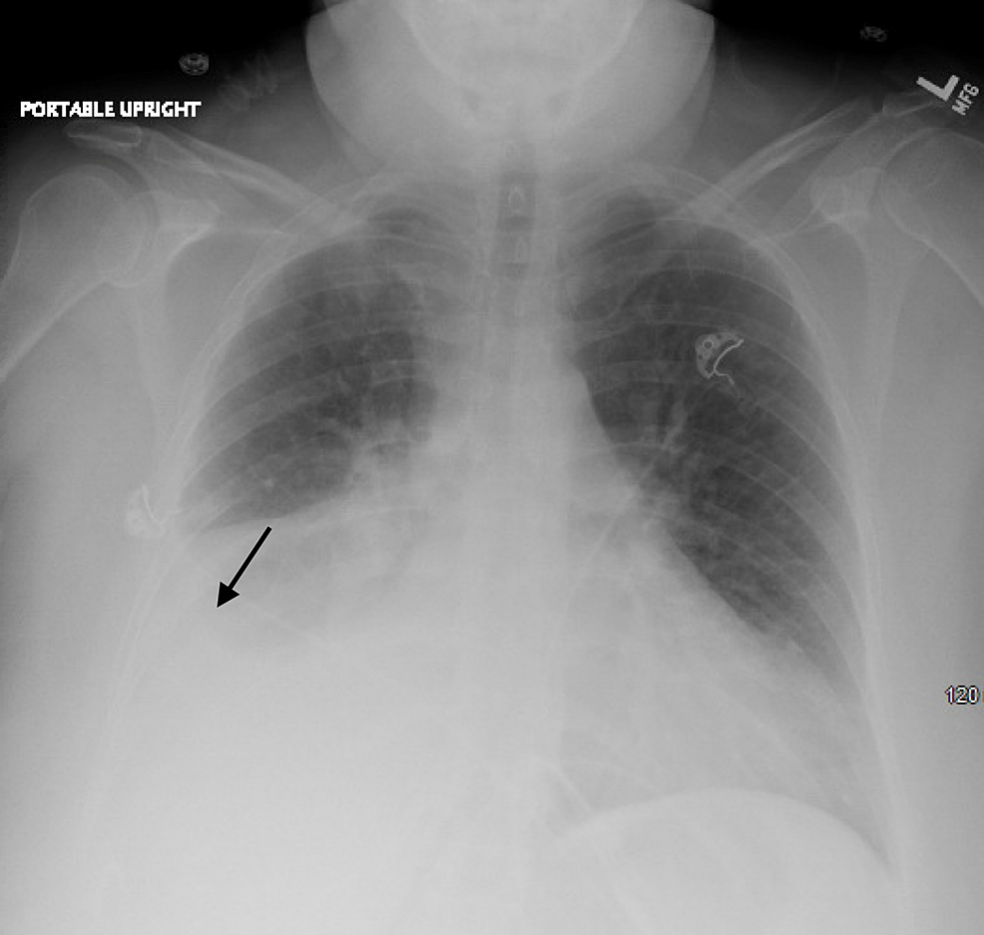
Portable chest X-ray on initial presentation showing massive right-sided pleural effusion with underlying airspace disease.
